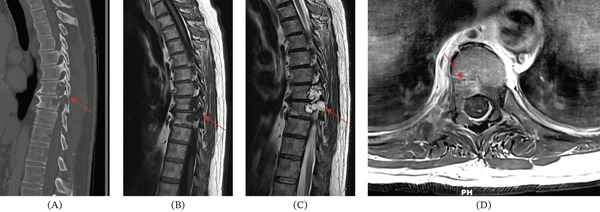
These sagittal and axial images further show the dominant T8–T9 lesion. (A) Panel A shows a lytic appearance on CT (red arrow). (B) Panel B shows low signal on noncontrast T1 MRI (red arrow). (C) Panel C shows T2 hyperintensity (red arrow). (D) Panel D shows moderate contrast enhancement in the anterior portion of the lesion (red arrow). These features are radiologically consistent with chordoma.
